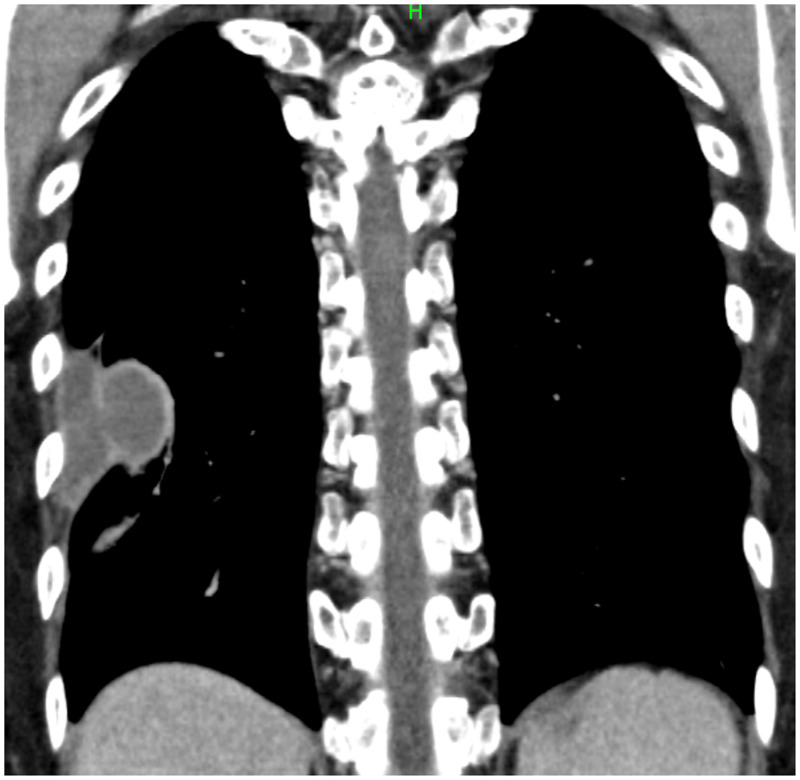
Coronal section of enhanced chest computed tomography. Encapsulated and peripherally enhanced low-attenuation mass adjacent to the posterolateral chest wall was observed. Note that the lower outline of the mass connects smoothly to the chest wall (extra-pleural sign), suggesting extra-pulmonary lesions. Pleural effusion was not detected.
